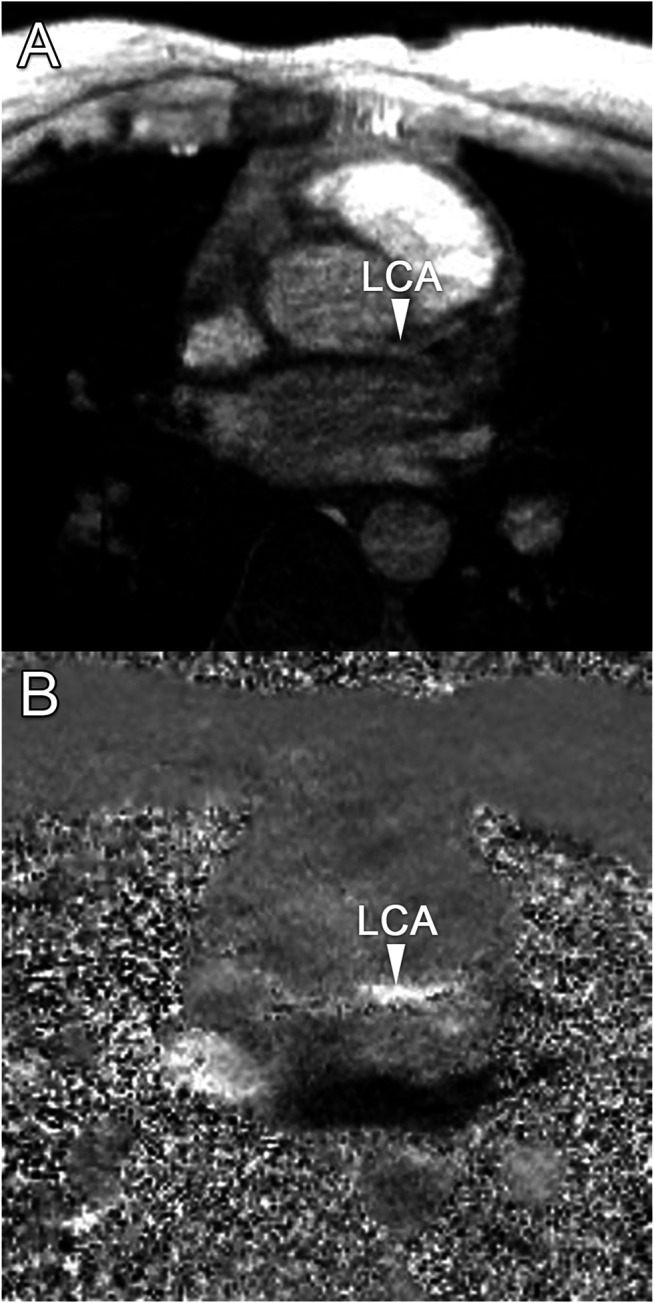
(A) Transversal 4D flow MRI magnitude image and (B) phase image showing velocities in right-left direction during mid-diastole. Arrows indicate the location of the left coronary artery, where velocity signal can be observed.
